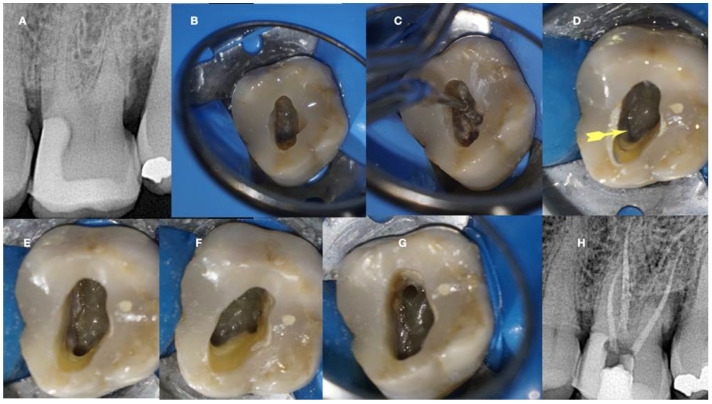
Calcified maxillary molar negotiation (A). Preoperative radiograph of the maxillary molar with no detectable canals (B). A clinical view of the access cavity reveals a completely calcified chamber (C). Clinical view of the ultrasonic troughing of the pulp floor. Notice that the pulp floor color is grey (D). Clinical view of the axial wall floor junction developmental line (yellow arrow). The canal entrances in a maxillary molar always originate from this line (E–G). Clinical image of the shaped canals. Notice that the location of the canals in a calcified maxillary molar is always lying along the wall–floor junction developmental lines (H). Postoperative radiograph of the calcified maxillary molar treatment (clinical images and radiographs courtesy of Dr. Chaniotis Antonis).
